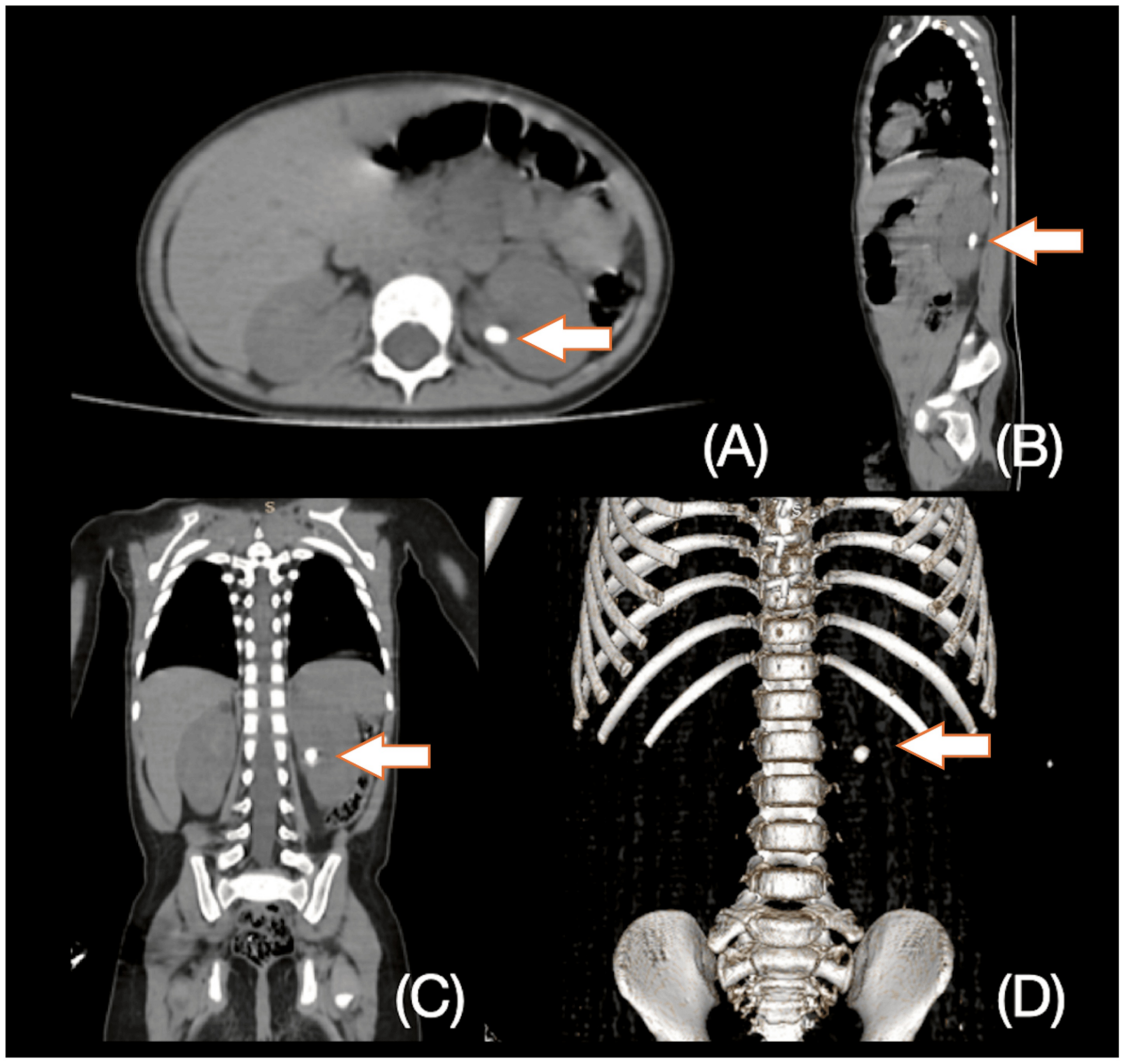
CT scan of the stone disease in the left kidney. A) Axial image, B) sagittal image, C) coronal image, D) 3D reconstruction.
